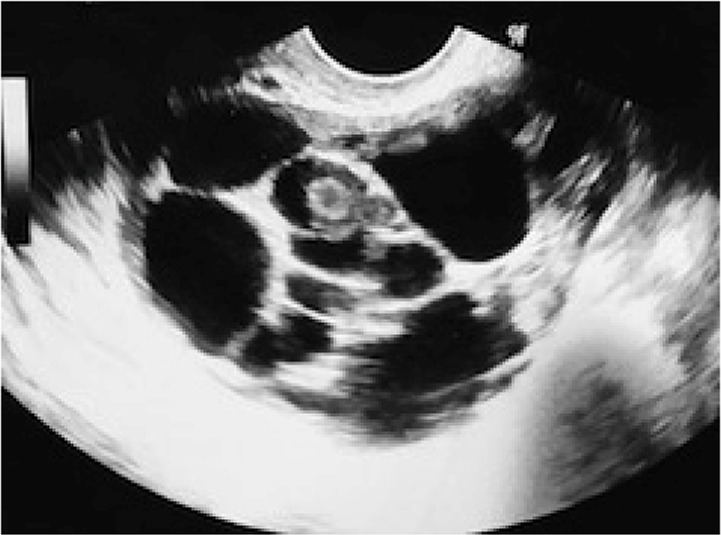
Transvaginal ultrasound image of the ovary before stimulation
